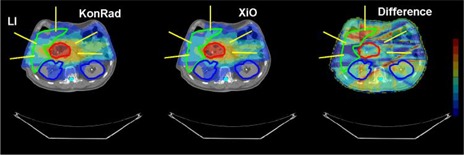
Beam arrangement and dose distribution of the liver case from the KonRad (left) and XiO (middle) systems. The beam directions are indicated as yellow lines on a transverse CT slice through the isocenter. The contours of the PTV is shown in red, the contours of liver, kidneys and spinal cord in green, blue, and cyan, respectively. The dose distribution for the two plans is shown as a color wash overlay. The right panel shows the dose difference between the two plans
